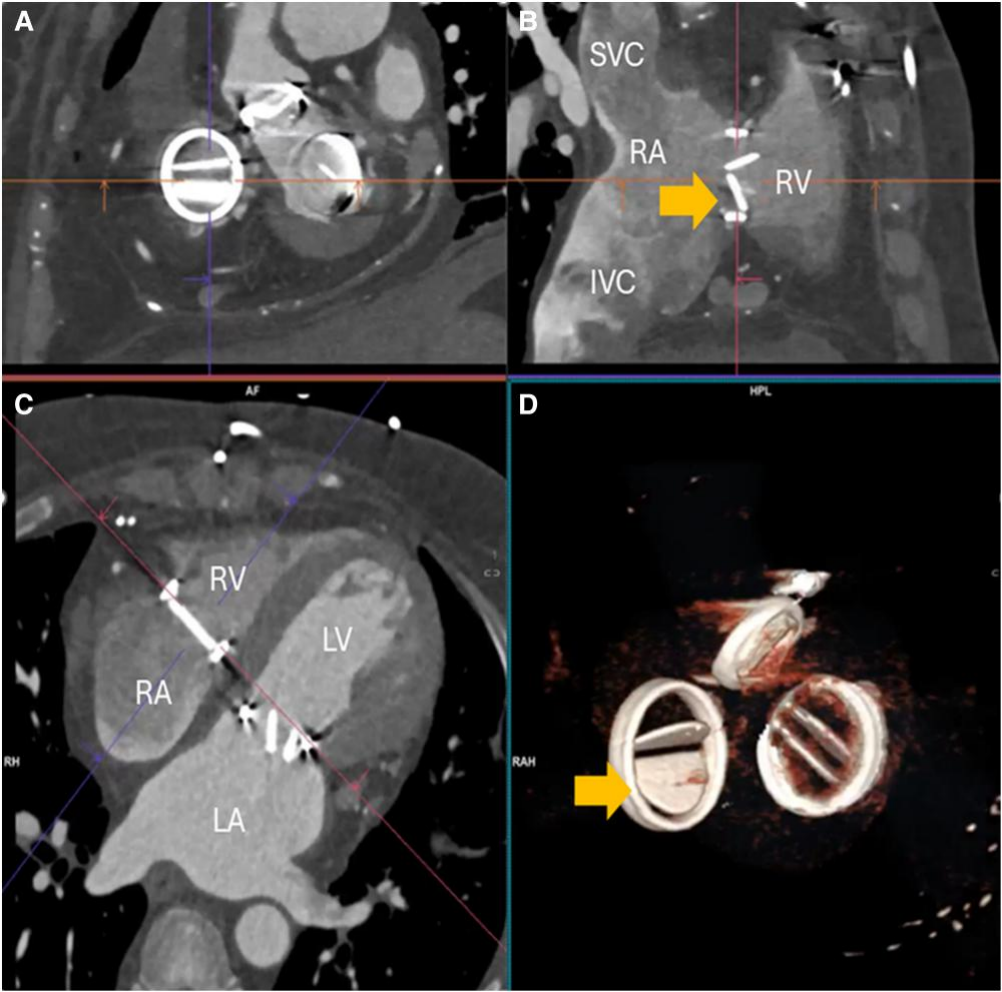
Contrast-enhanced gated cardiac computed tomography at diastole post-thrombolysis and percutaneous intervention and on therapeutic anticoagulation. The anterior leaflet was open, but the posterior leaflet was now immobile at diastole (arrow on *Panels B* and *D*).
